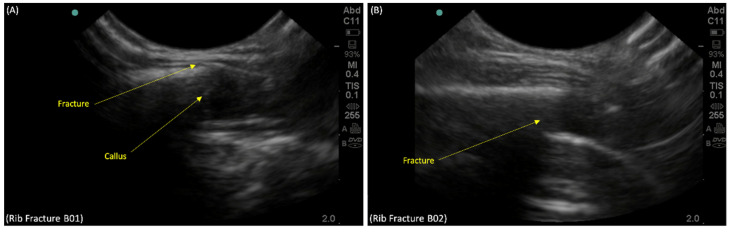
Point-of-care-ultrasound of the right fourth rib on longitudinal view showing: (A) a disruption in the callus of the old fracture on the right fourth anterior rib (B01), suggesting a refracture of the right fourth anterior rib and (B) cortical disruption (B02) of the right posterior fourth rib on longitudinal view using POCUS, suggesting a new fracture as reported in the chest radiographs.
